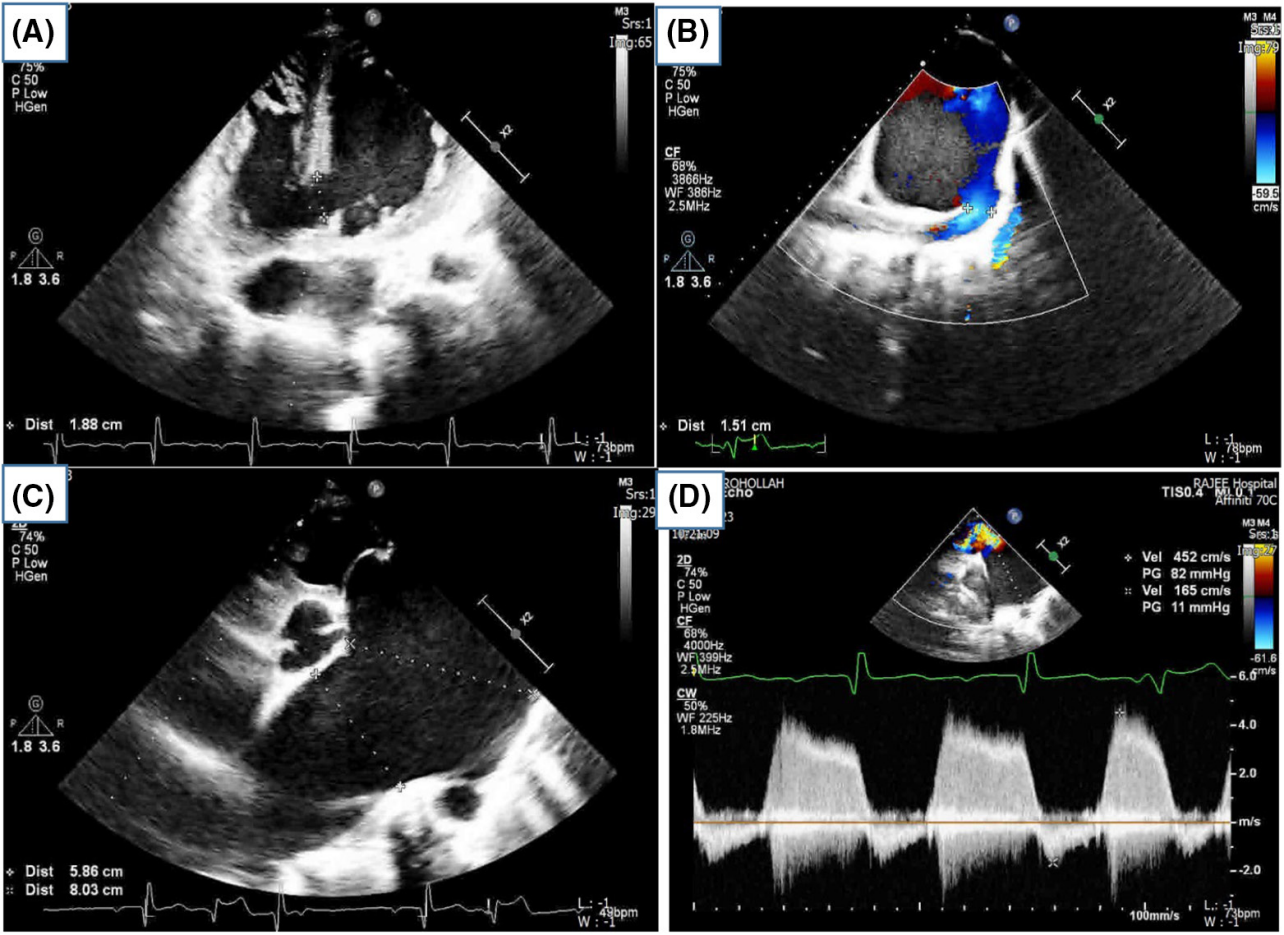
Patient's echocardiographic findings: (A) large high muscular ventricular septal defect (2.2 cm) with a bidirectional shunt in trans‐thoracic echocardiography (four chamber view); (B) large patent ductus arteriosus (1.5 cm) with a bidirectional shunt in trans‐thoracic echocardiography (short‐axis view); (C) aneurysmal main PA (8 cm), RPA (5.3 cm), and absent LPA in trans‐thoracic echocardiography (short‐axis view); (D) severe PH (mean PAP = 87 mmHg) in trans‐thoracic echocardiography (short‐axis view).
